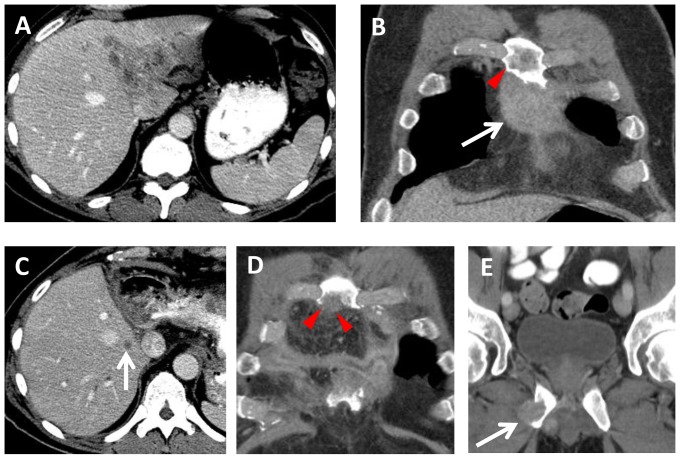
A 57 year old male with BAP1 mutated intrahepatic CCA. Baseline (A) axial and (B) coronal contrast-enhanced CT images demonstrate (A) a 6.9×5.3 cm liver mass involving segments I, II, and IV, and (B) a 5.6 cm sternal metastasis (arrow). Note the sternal metastasis does not extend to the level of the right 3rd rib (arrowhead). Extended left hepatectomy and resection of the sternal metastasis was performed. (C, D) Seven weeks later, contrast-enhanced CT images show a new metastasis in the remnant liver (arrow), and a new 2 cm recurrence (arrowheads) involving the sternum adjacent to the right 3rd rib. (E) Three months later, there is a new 2.2 cm metastasis (arrow) involving the right inferior pubic ramus.
